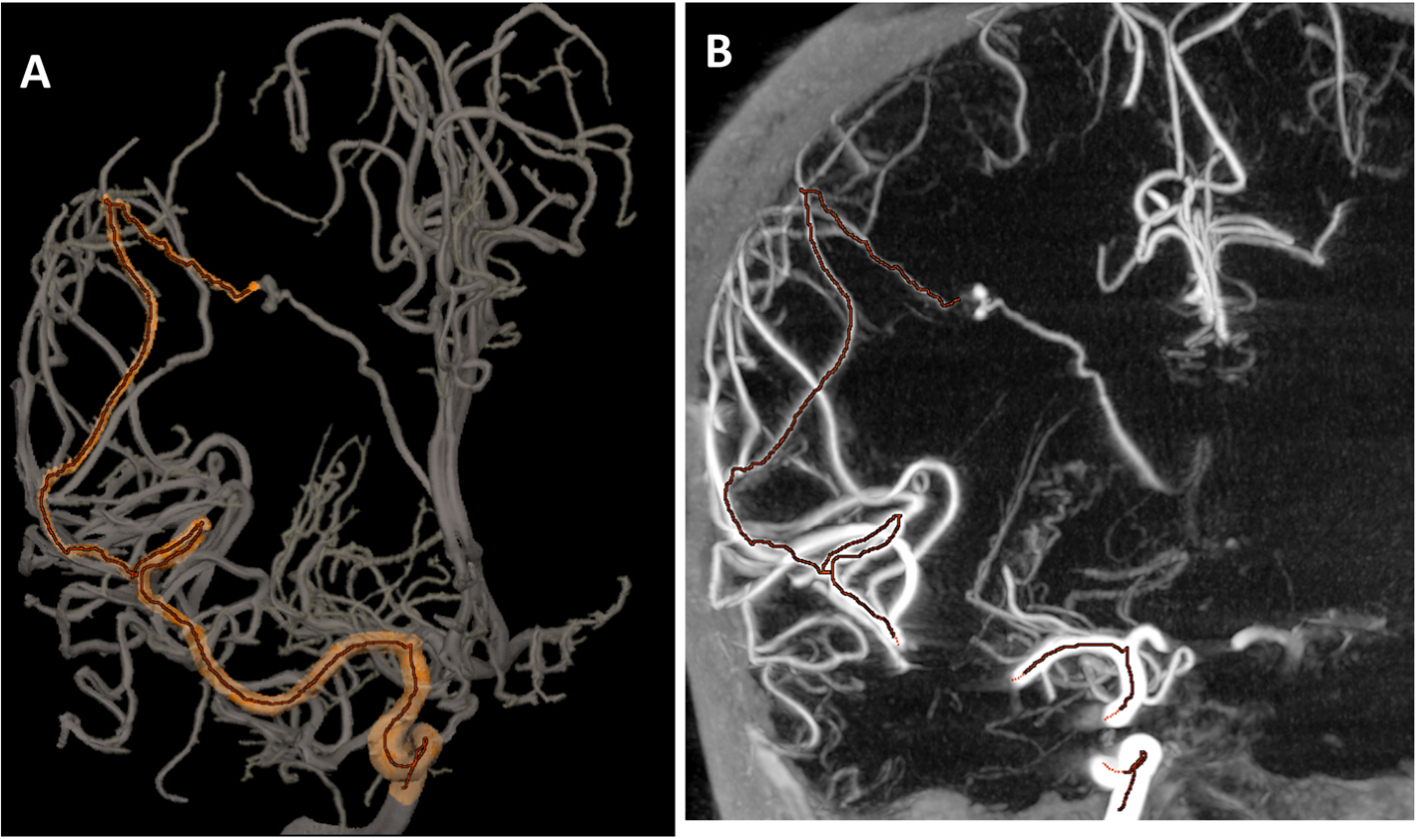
Retrospective review of a distal aneurysm. Virtual catheterization software was also evaluated for distal embolization, such as in a context of mycotic aneurysm. The software was used to create a 3D roadmap based on the CBCT (a). The accuracy of the 3D roadmap was assessed using the virtual catheterization trace on the cross-sectional views (b)
